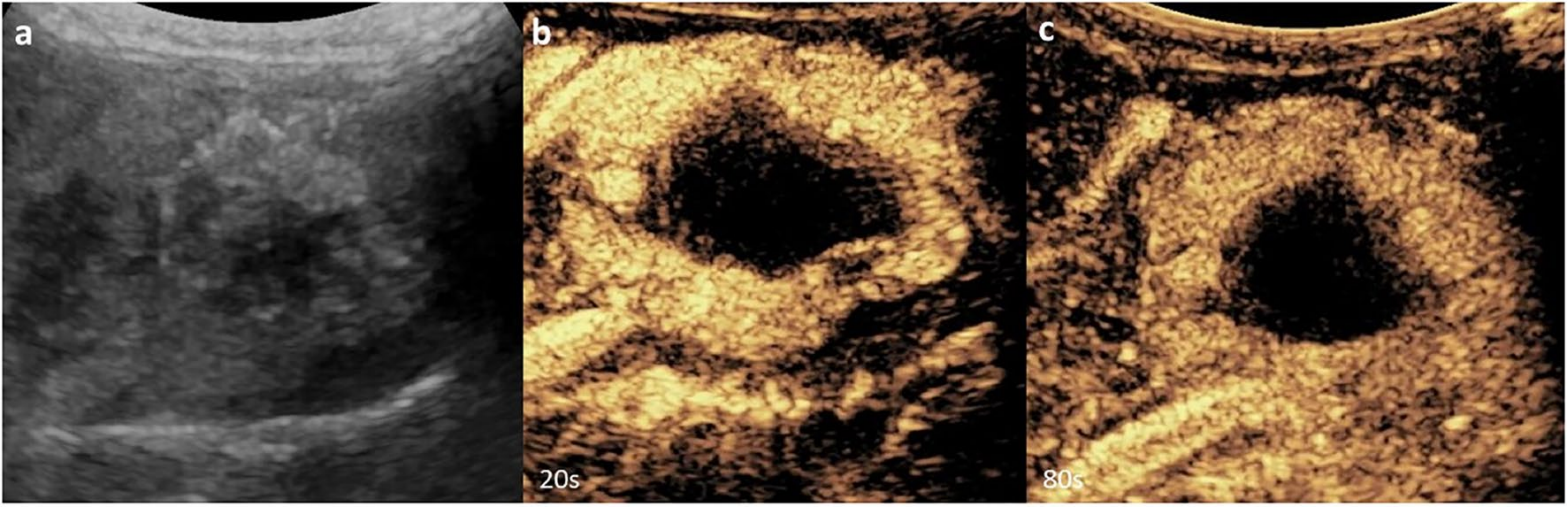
16-week-old female. Contrast ultrasound of the liver, transverse right upper quadrant scan. Post-natal evaluation of a pre-natally diagnosed congenital hemangioma showing a grayscale appearance of a heterogenously echogenic lesion with poorly defined borders and central hypoechogenicity; and post contrast images showing b arterial and c late venous phase enhancement, with persistent hypoenhancement centrally but no washout
